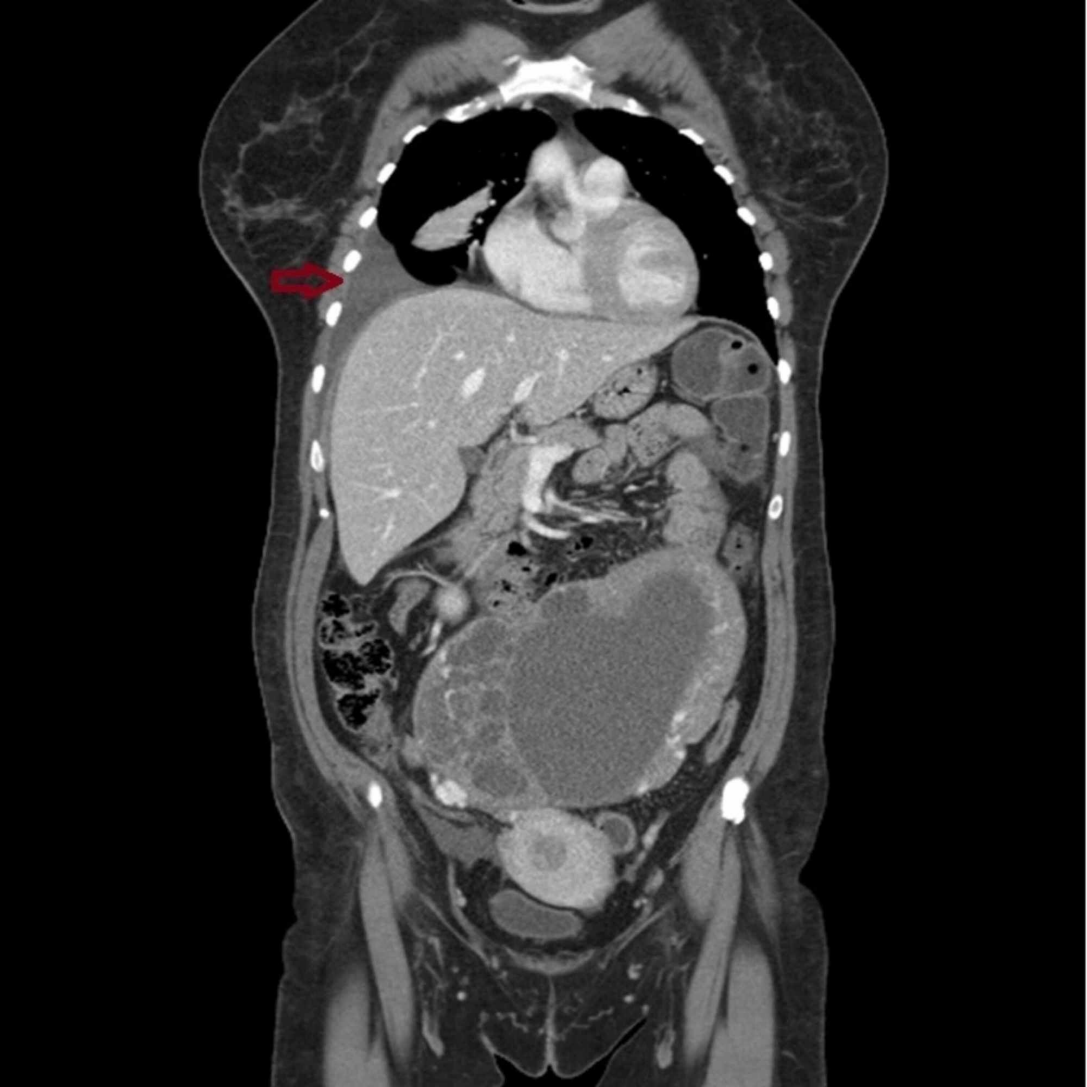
CT scan, coronal view. A heterogeneous pelvic mass of 17 cm diameter without lymphadenopathies was noted. The arrow points to the pleural effusion. A scanty amount of free peritoneal fluid was observed (not visible in this cut).
